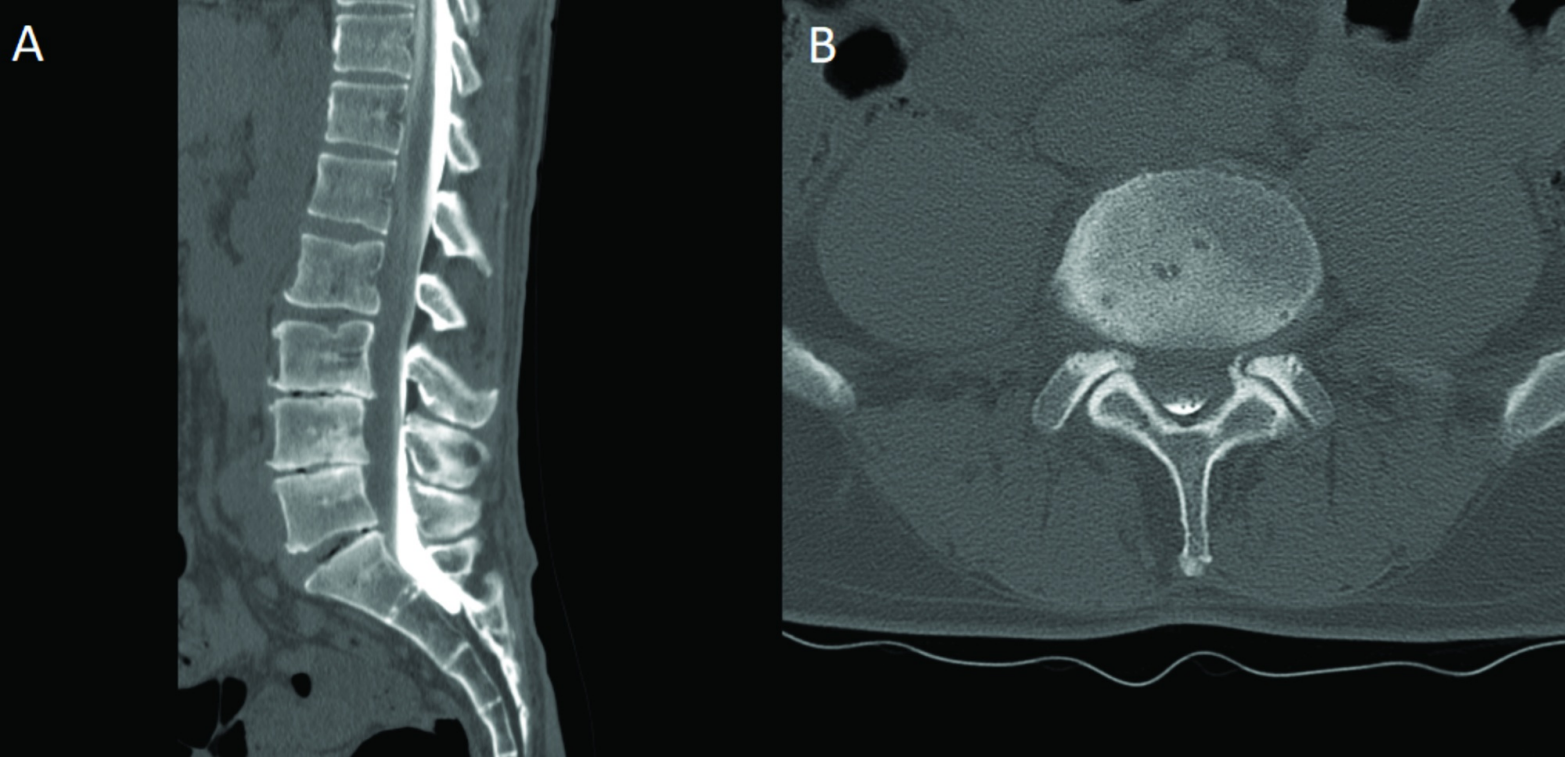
CT myelogram. A) Sagittal cut demonstrating multi-level spondylosis with collapse of disk height most pronounced at L3-L4 and L4-L5; B) Axial cut at the L4-L5 level demonstrating difficulty in determining central or neuroforaminal stenosis using this technique CT: computed tomography
